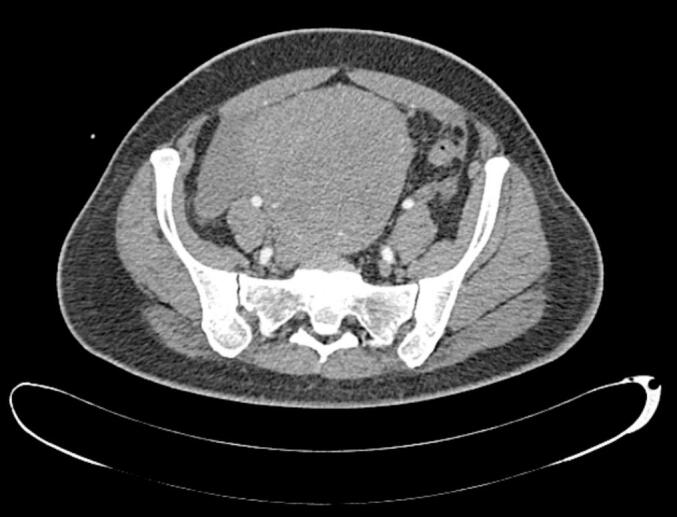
Computed tomography (CT) image of uterus demonstrated fibroid uterus with sinister uterine mass and haemoperitoneum.
